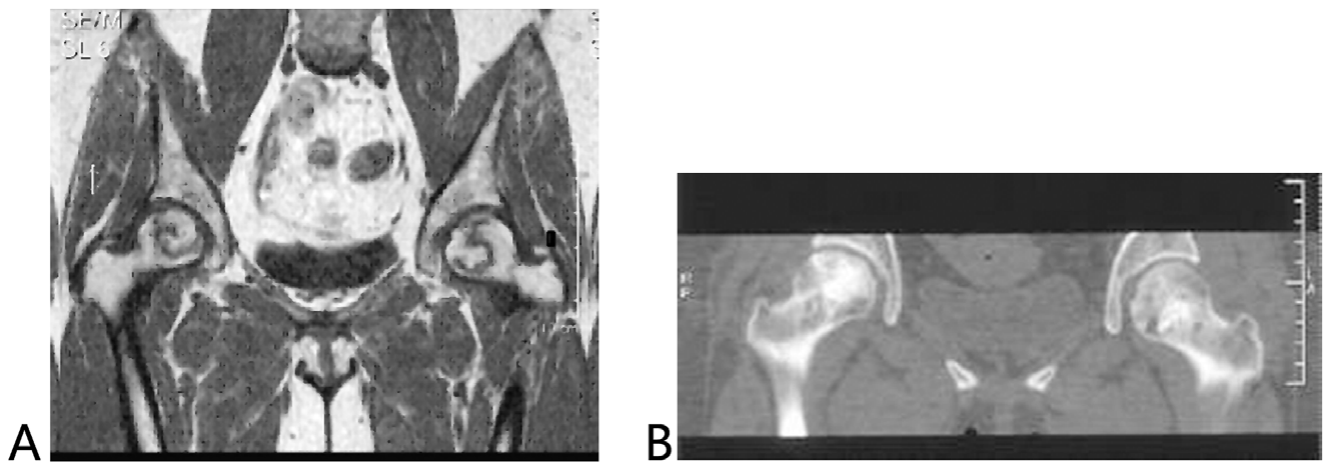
A 30-year-old patient with ONFH after SARS. A: Preoperative magnetic resonance image showed ARCO stage IIc/CJFH Type L3 (the right hip) and ARCO stage IIb/CJFH Type C ONFH (the left hip); B: This Computed tomography scan was performed five years after being treated with impacted bone grafting with the addition of rhBMP2 and showed the complete repair of the osteonecrotic region, the amount of sclerotic bone was more improved and the articular surface remains intact without collapse.
